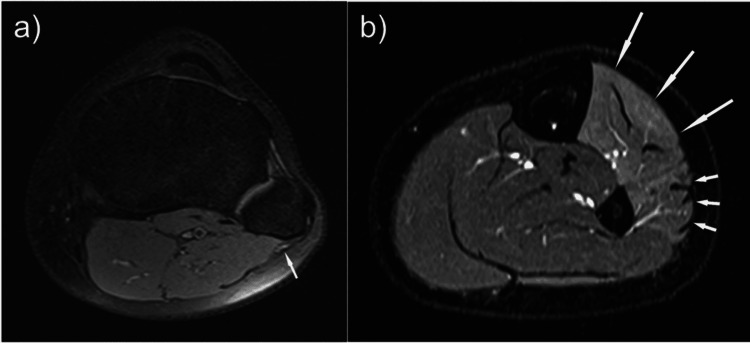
(a) Axial T2 fat-supressed MRI slice of patient 2’s left knee demonstrating swelling of the common peroneal nerve at the fibula head. (b) Axial T2 fat-suppressed MRI slice of the mid-calf demonstrating oedema of the anterior (long arrows) and peroneal (short arrows) compartments.
